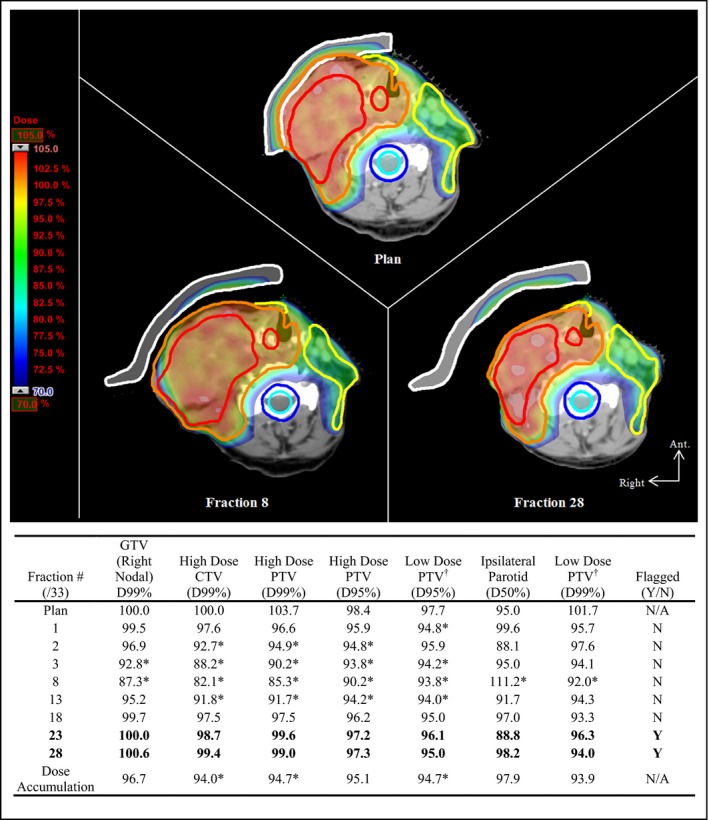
Localized change in body contour adjacent to the high‐dose PTV exhibited by Patient 1. Top: pretreatment CT and original plan with digitally rendered bolus (upper), synthetic CT image at fractions 8 (lower left) and 28 (lower right), and recalculated original plan with physical bolus placed on the external surface of the mask. 70% of the prescription dose (0.70 × 70 Gy = 49 Gy) approximately corresponds to the 3% major violation overdose of the spinal cord (49.4 Gy). Bottom: dose parameters calculated for major and minor violation criteria on fractions with CBCT acquisition. Entries are expressed as a percentage of planning objective or planned parameter value (see Table 2). Bold entries indicate fractions flagged by the protocol. (Contours: red — high‐dose GTV, orange — high‐dose PTV, yellow — low‐dose PTV, cyan — spinal cord, blue — spinal cord with margin, white — bolus). *Clinically significant deviation, according to the major/minor violation criteria (only those parameters violating Table 2 criteria are shown). ^†^Low‐dose PTV volume excludes the high‐dose PTV volume.
